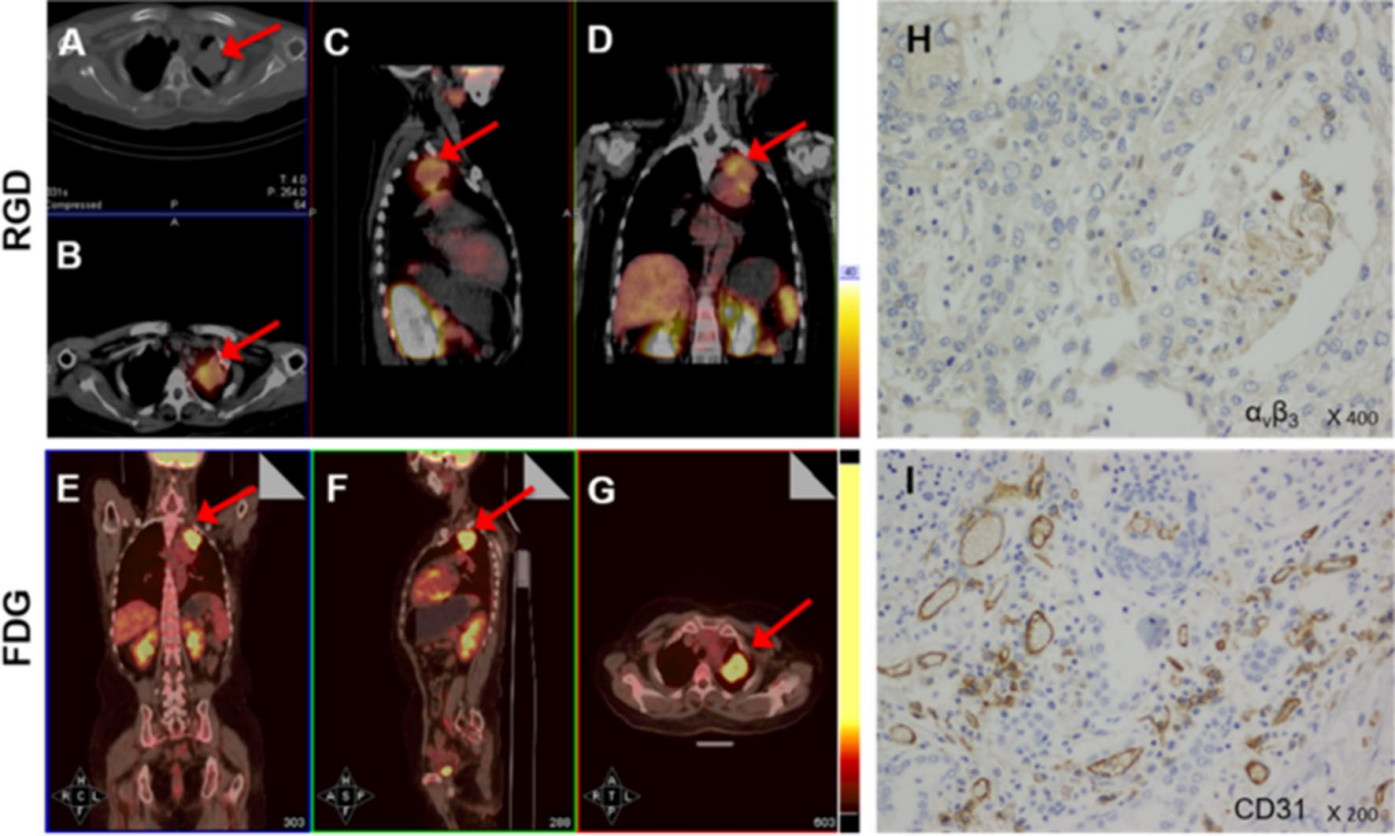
[^99m^Tc]Tc-Galacto-RGD_2_ SPECT/CT showed RGD-avid lesion in a female patient with squamous cell carcinoma. a–d RGD imaging showed focal uptake in the left lung. e–g FDG-avid lesion shown in the left upper lung. h, i IHC showed a moderate α_v_β_3_ expression in the tumour cell membrane, and a high density of neovascularization with high expression of CD31
